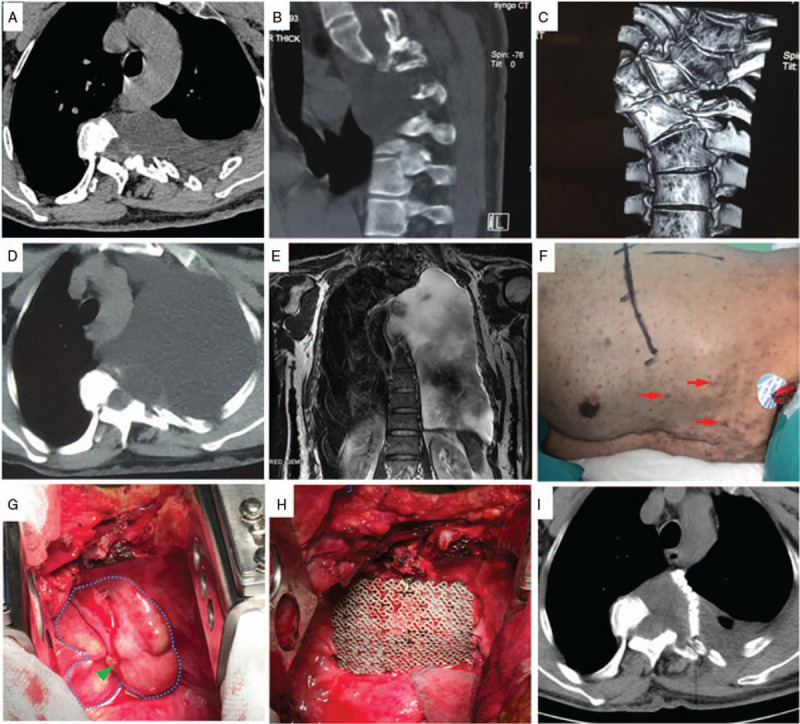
Intrathoracic meningocele in patient 3. Chest computed tomography (A) and thoracic spine three-dimensional computed tomography (B, C) shows a homogeneous cystic mass with a thin wall in the left paraspinal space with scoliosis before rupture of the cystic. Chest computed tomography (D) and coronal chest MRI show a large number of pleural effusion after the rupture of the cystic. Disseminated cafe ´-au-lait spots and subcutaneous nodules (red arrow) on the chest and abdomen of the patient (F). Intraoperative image (G) shows a meningocele with a rupture of the cystic wall (green arrowhead). After repaired the cystic wall, a piece of titanium mesh was applied to prevent meningocele expansion (H). Postoperative chest CT (I) show a small residual pseudo meningocele and mesh repair in place with little pleural effusion.
